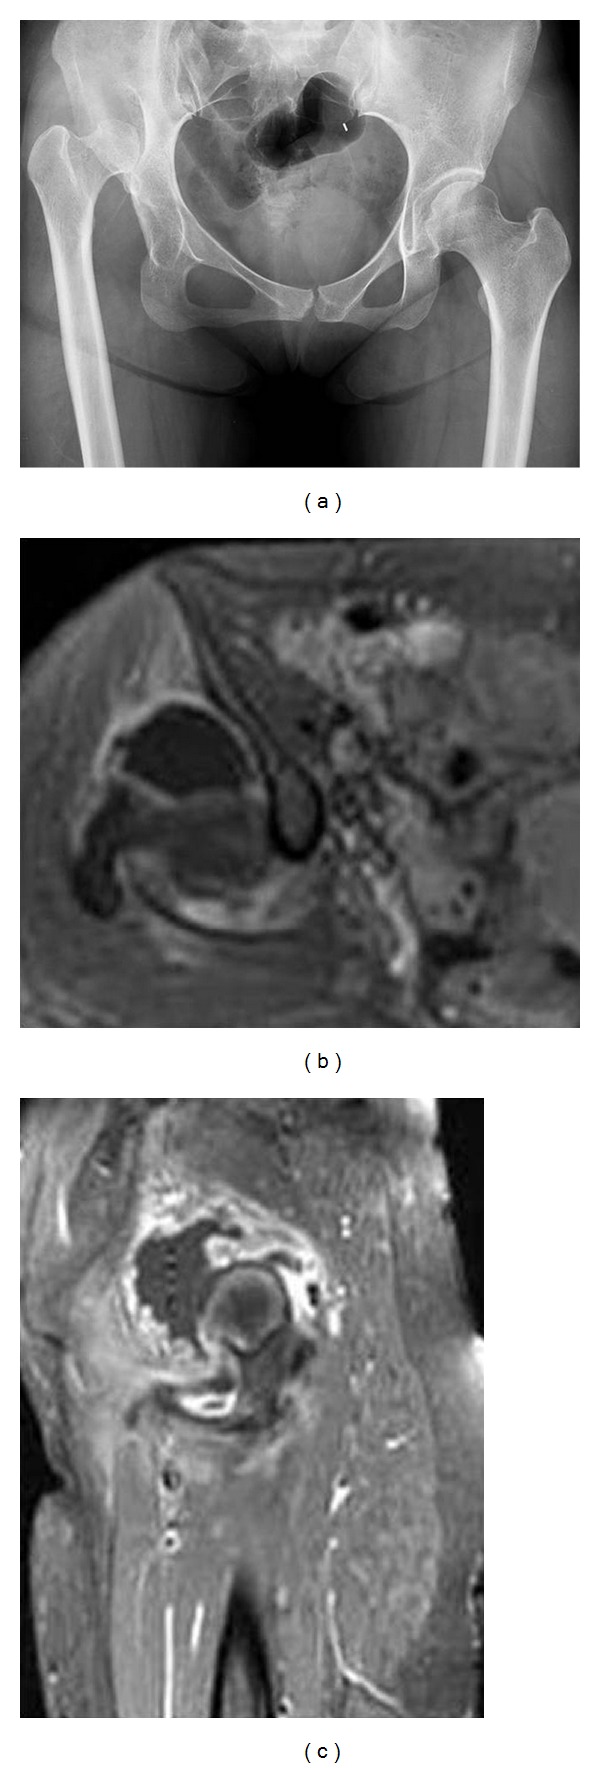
(a) Preoperative hip anteroposterior radiograph of a 28-year-old woman shows right hip high dislocation, a small femoral canal, and a pseudoacetabular joint. (b) and (c) Preoperative axial and sagittal T2 weighted enhanced MR images of the right hip joint show an inflammatory reaction with huge joint effusion and synovial thickening.
